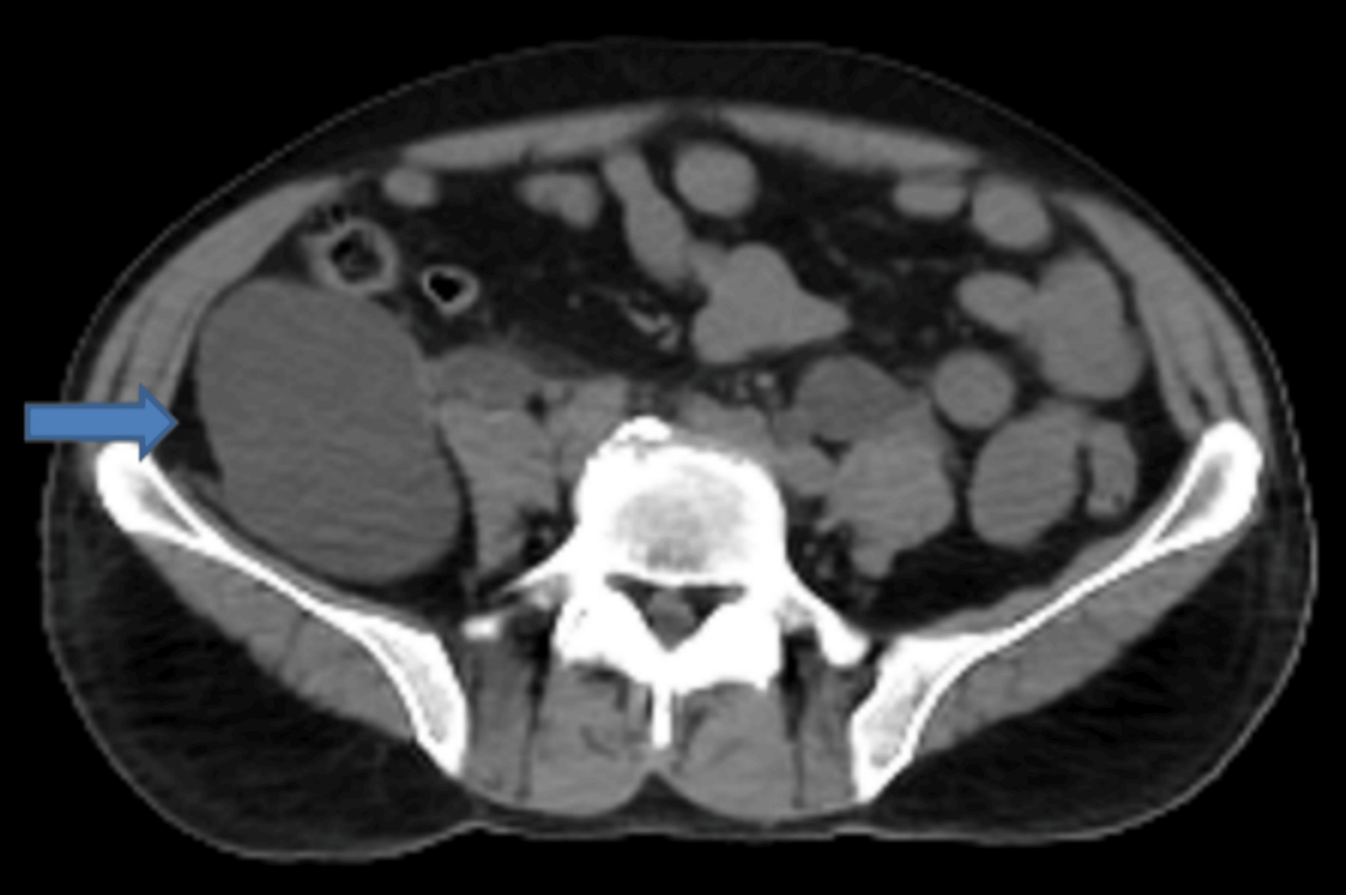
Non-contrast CT of the abdomen (axial view) showing an encapsulated fluid collection in the right lumbar region (blue arrow). The collection appears to be inseparable from the right ureter with moderate dilatation of the right collecting system.
